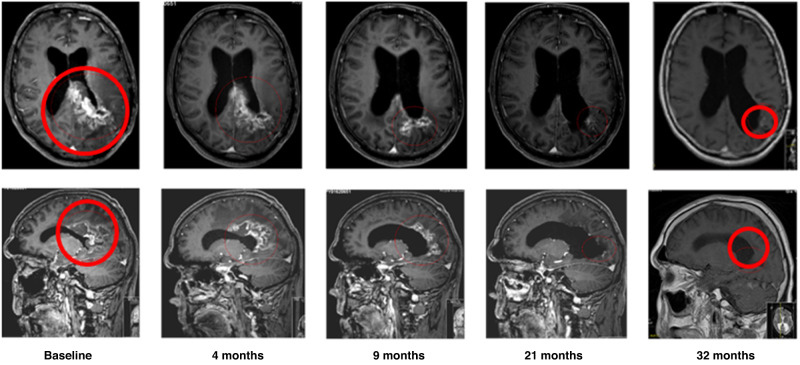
MRI scans over time of a patient with partial response. The patient showed a sustained tumour regression (partial response according to RANO criteria) over time for 3 years on monotherapy with 2-OHOA (500 mg BID, cohort 2). MRI transverse (upper scans) and sagittal (lower scans) brain images show the glioblastoma (red circle) reduction, which was determined to be ca. −93% of the initial volume.
